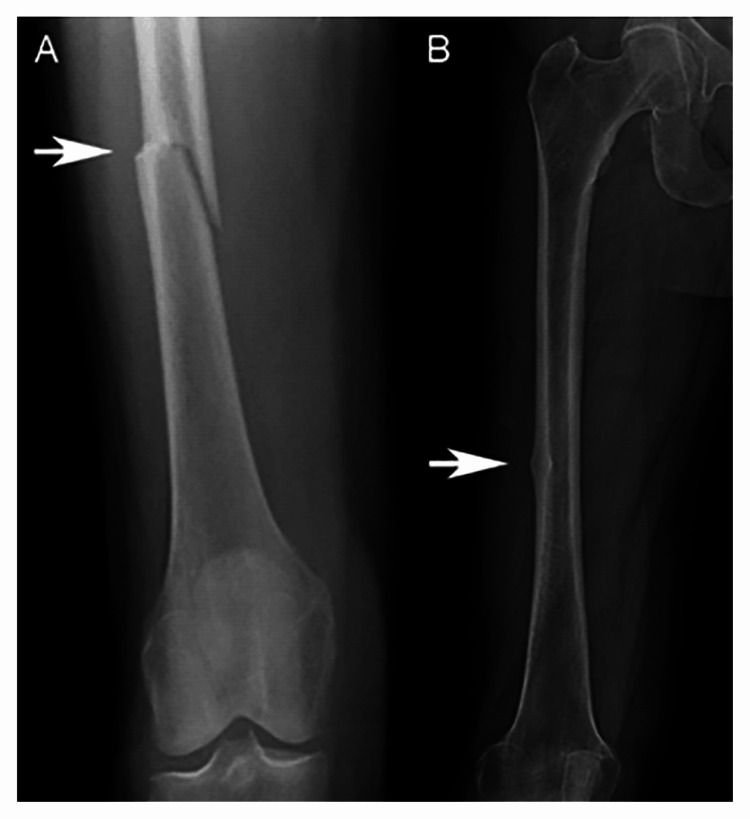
Radiographic views of an (A) atypical femoral fracture that occurred spontaneously in a woman after six years of treatment with a bisphosphonate and (B) incomplete atypical femoral fracture with cortical thickening that presented as thigh pain. Source: Khan AA, Kaiser S. Atypical femoral fracture. CMAJ. 2017, 89:E542 Open access journal under a CC-BY license
